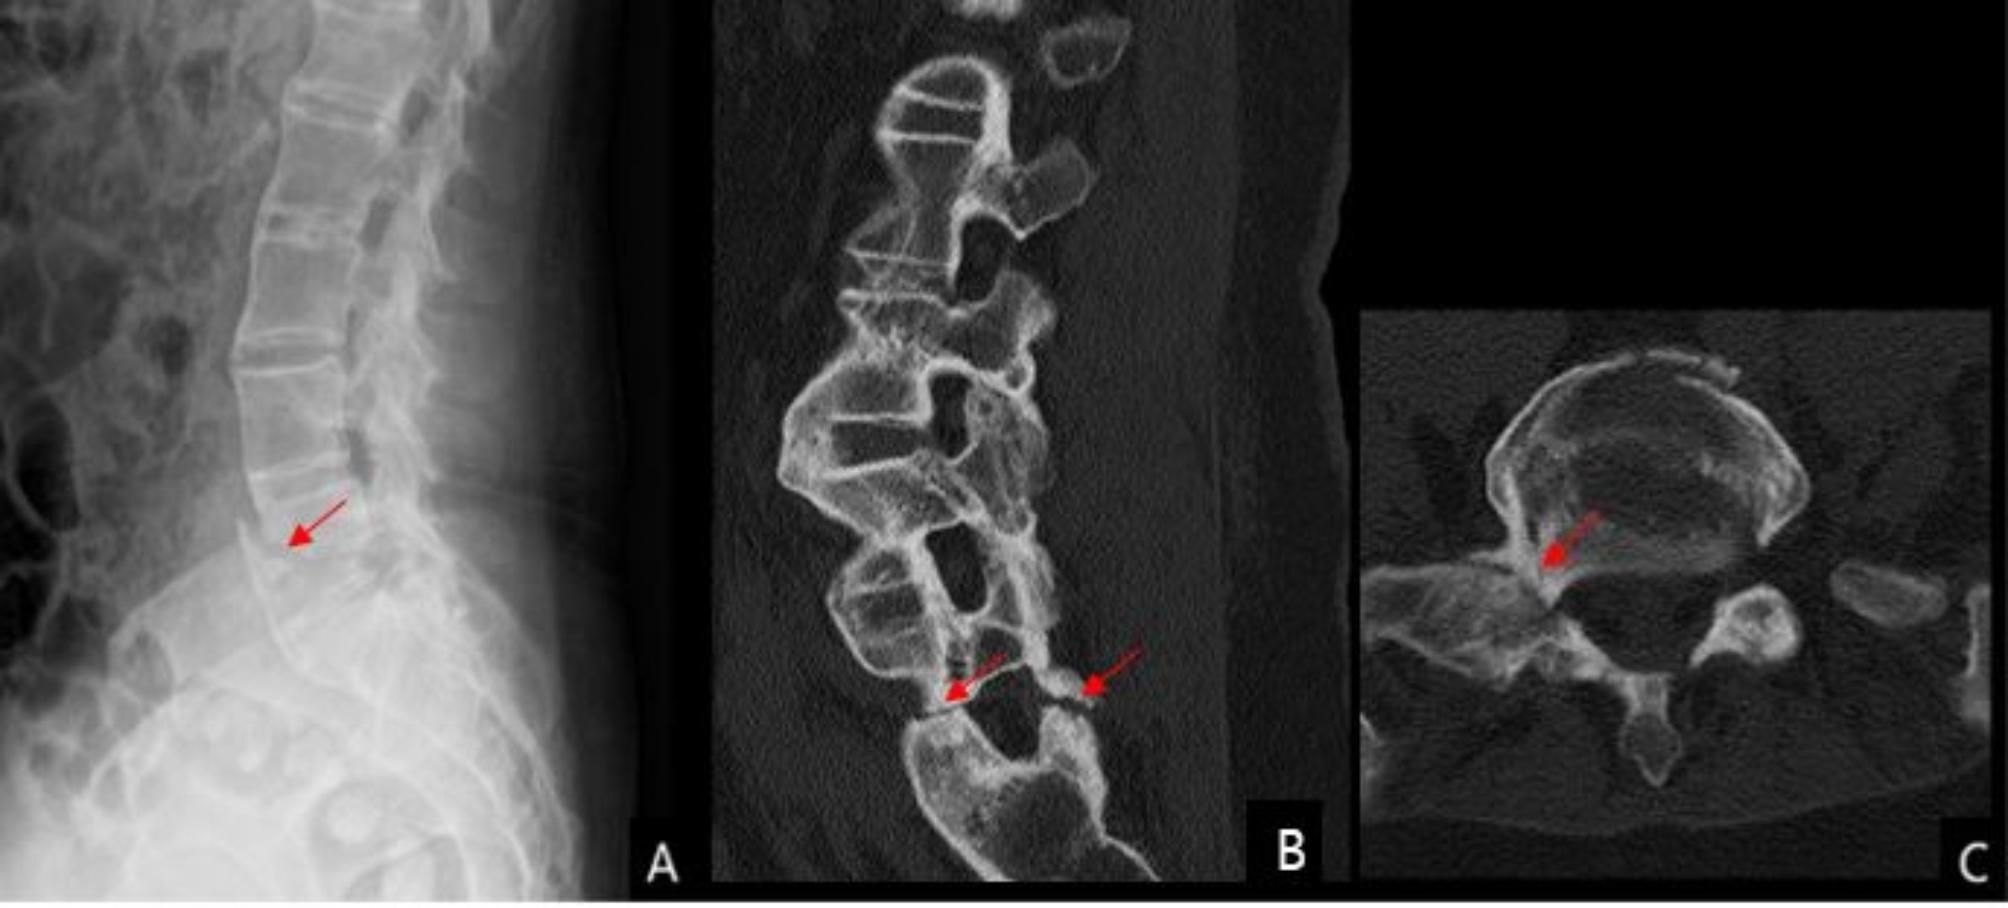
A) Simple lateral radiograph reveals discontinuity of ossified ligament and displaced L5 fracture. B & C) Computed tomography (CT) scans show vertebral body and posterior element fractures (arrows)
